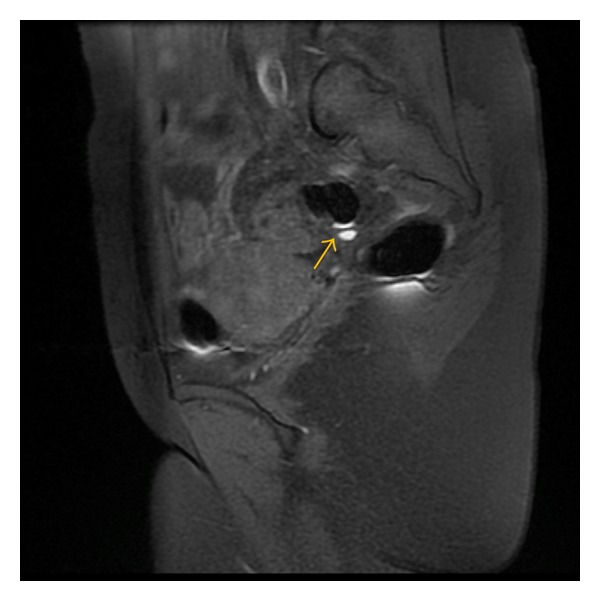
Sagittal T1-weighted fast SE image with fat suppression (TR/TE = 580/7.7) shows small hemorrhagic focus (arrow) in the rectal wall.
